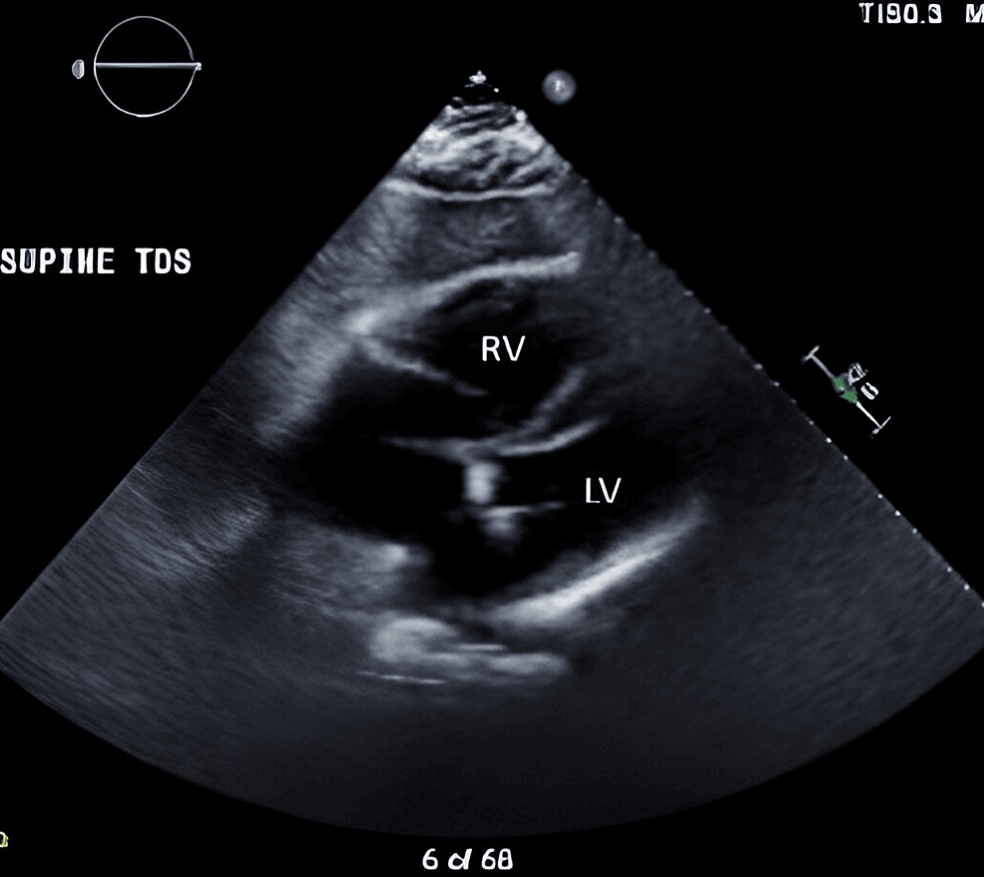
Echocardiography while the patient was supine Left ventricle appears normal in size with low normal systolic function; right ventricle is dilated with reduced function
